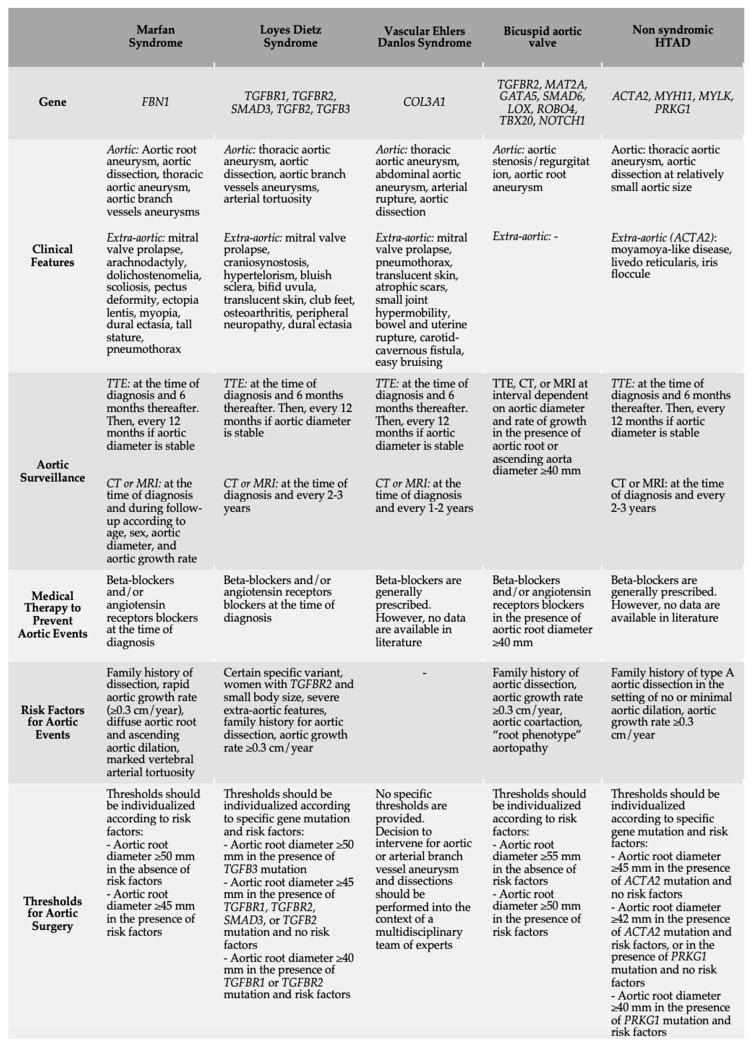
Clinical and genetic characteristics, risk factors for acute aortic events, surveillance, medical therapy, and recommendations for aortic surgery according to each HTAD. Aortic surveillance and thresholds for aortic surgery are those proposed by the 2022 American College of Cardiology/American Heart Association guidelines for the diagnosis and management of aortic disease [5]. Abbreviations: CT, computed tomography; MRI, magnetic resonance imaging; HTAD, heritable thoracic aortic disease; TTE, transthoracic echocardiography.
